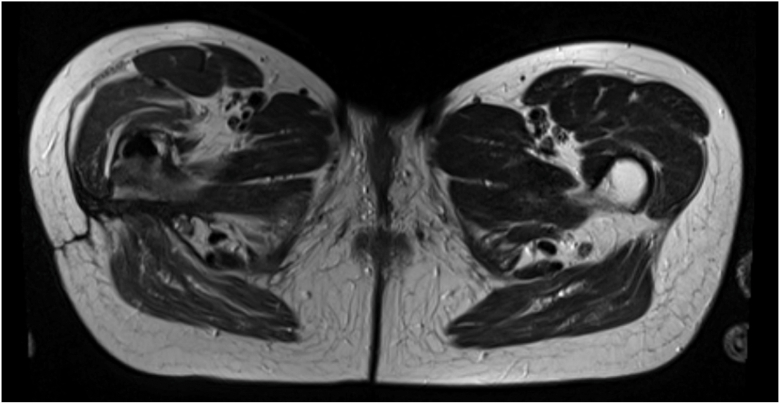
Axial view of an MRI with T2 weighting and TSE sequencing (Metal Artifact Reduction Sequence) at 1-year follow-up at the level of the previous pseudocyst in the right thigh. TSE, turbo spin echo.
